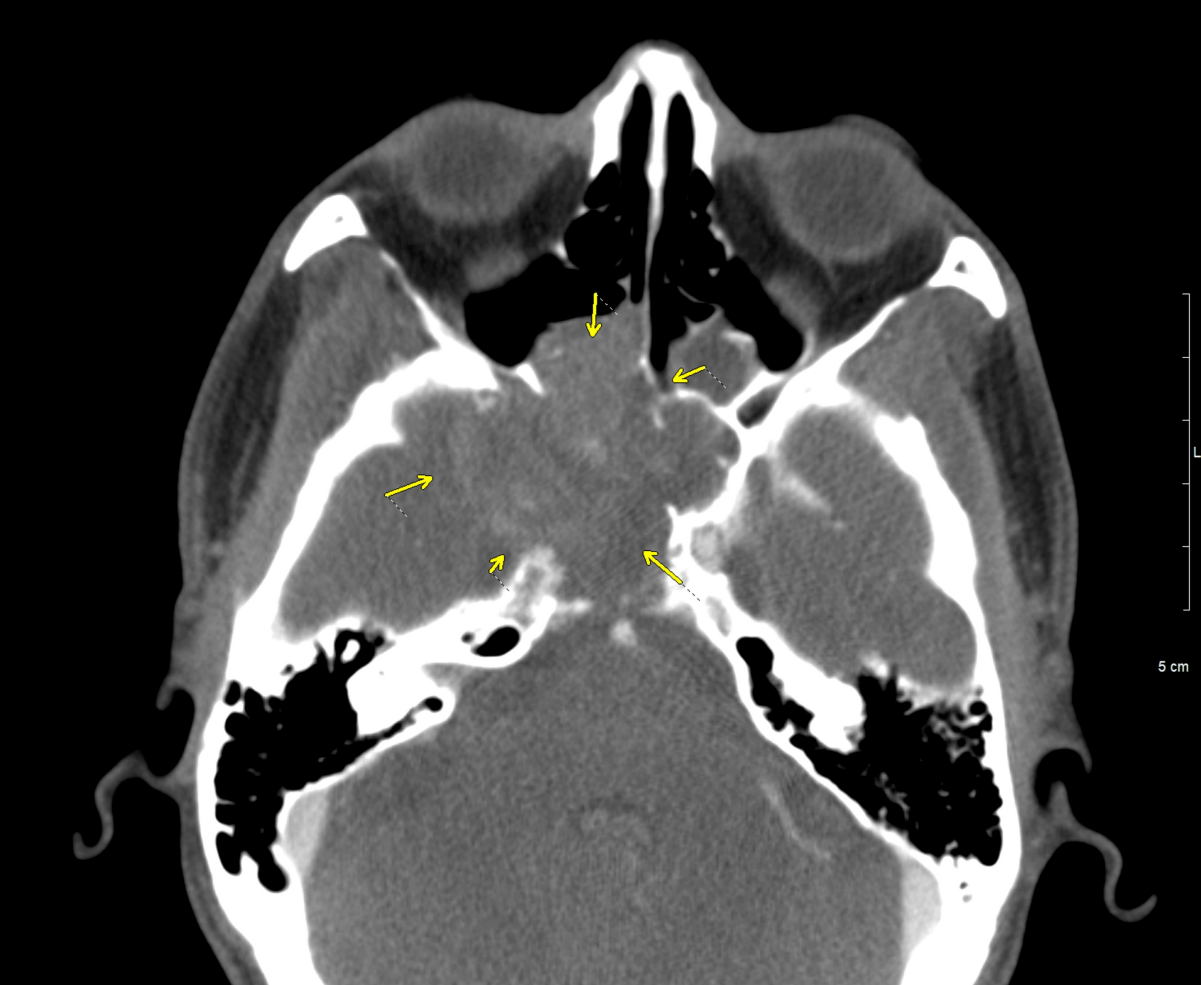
CT axial head showing large heterogenous mass (yellow arrows) centered within the ethmoid sinus with extension into right middle cranial fossa.
